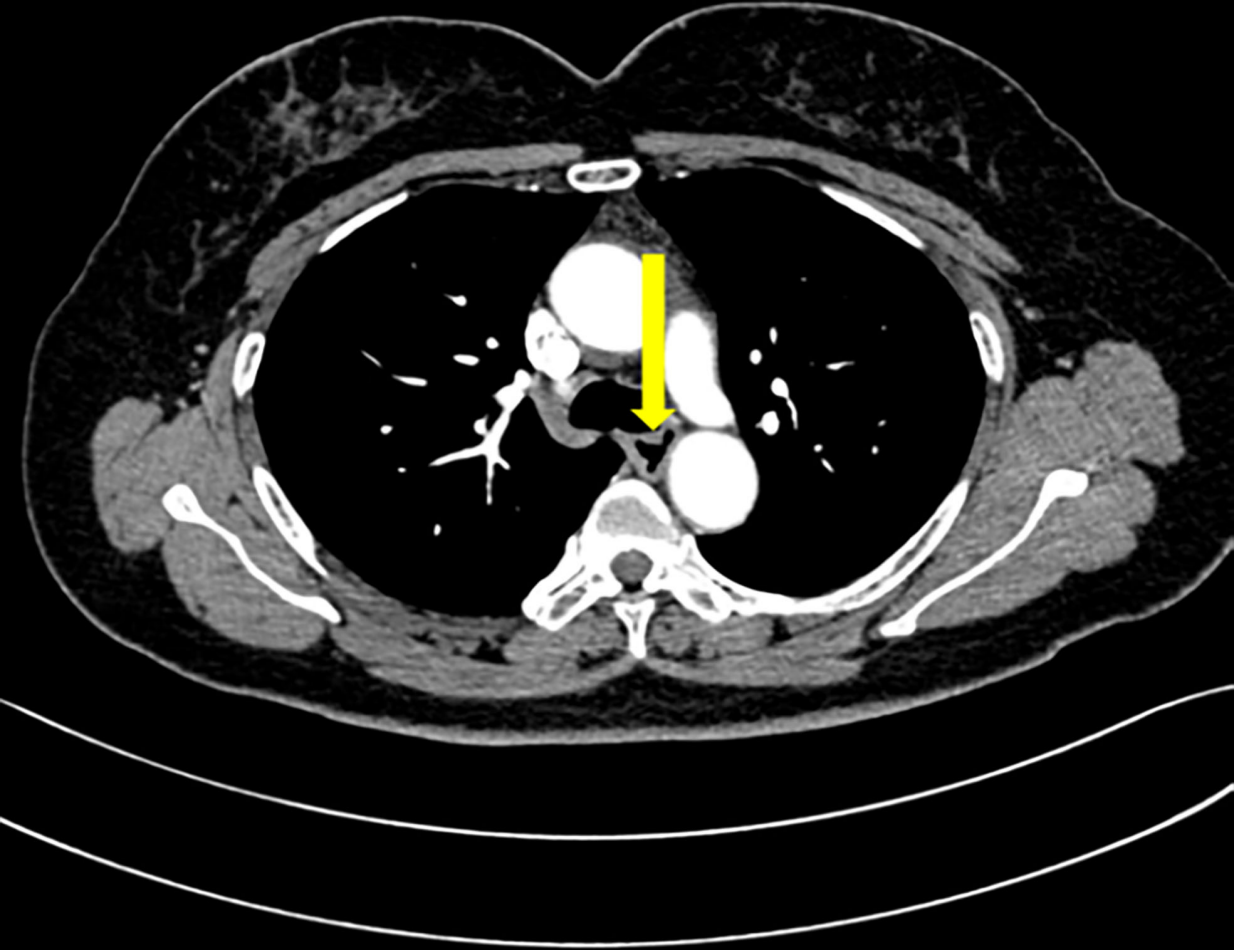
Enhanced chest CT. Enhanced chest CT showed that the wall of the upper and middle segments of the esophagus was slightly thickened and the lumen slightly narrowed.
